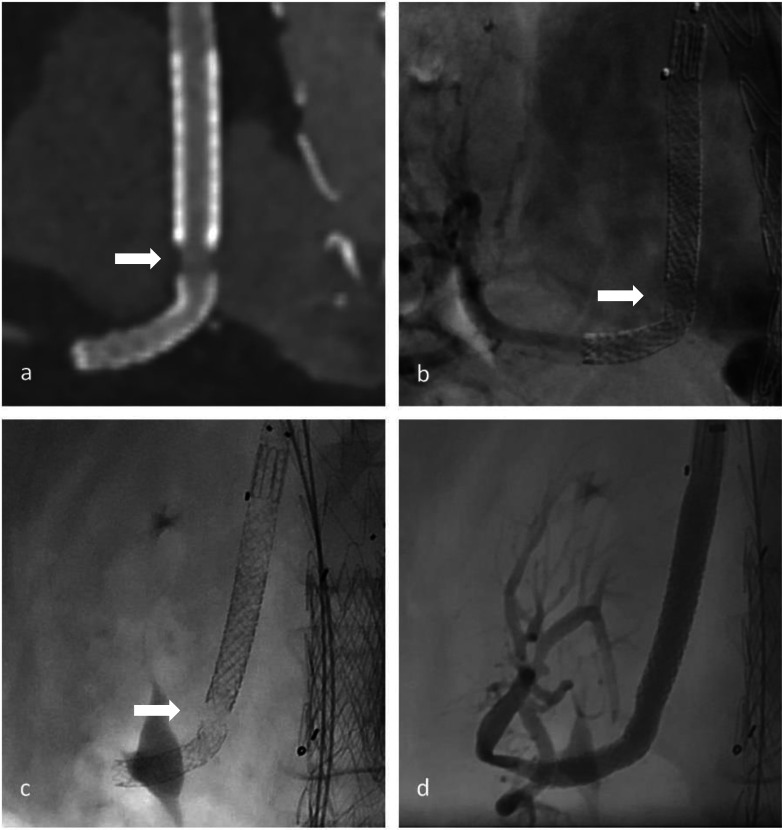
Complete separation of right renal BSG with endoleak directly diagnosed at CTA (a), during angiography (b, c) and after successful religning (d)
